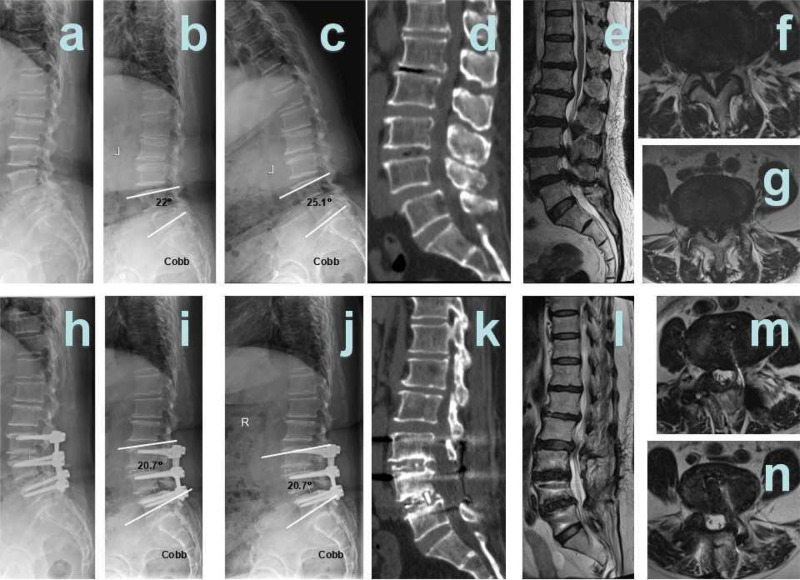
TLIF patient 2. (A) Pre-surgical lateral X-ray; (B) pre-surgical hyper-extension X-ray; (C) pre-surgical hyper-flexion X-ray; (D) pre-surgical CT showed L3/4, L4/5 spinal stenosis, L4 I° spondylolisthesis; (E) pre-surgical T2-weighted MRI sagittal plane showed L3/4, L4/5 spinal stenosis; (F) pre-surgical MRI showed L3/4 spinal stenosis; (G) pre-surgical MRI showed L4/5 spinal stenosis; (H) 12 months after surgery, post-surgical lateral X-ray; (I) 12 months after surgery, post-surgical hyper-extension X-ray; (J) 12 months after surgery, post-surgical hyper-flexion X-ray; (K) 12 months after surgery, post-surgical CT showed interbody fusion; (L) 12 months after surgery, post-surgical T2 weighted MRI sagittal plane; (M) 12 months after surgery, post-surgical MRI sagittal plane showed that L4/5 spinal stenosis was improved significantly; and (N) 12 months after surgery, post-surgical MRI sagittal plane showed that L5/S1 spinal stenosis was improved significantly.
